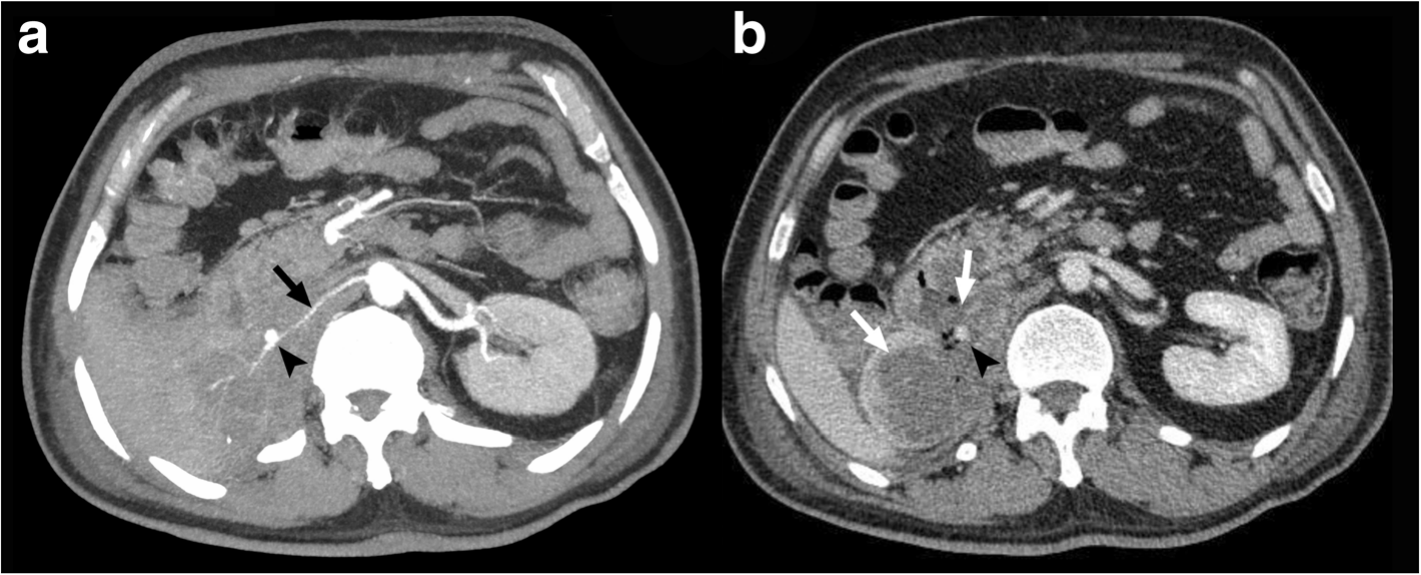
Maximum intensity projection (MIP) reconstruction (a) and thin slice (b) images of contrast-enhanced abdominal CT scan in the arterial phase show a small right renal artery (arrow in a) with a pseudoaneurysm (arrowhead in a and b), and a hypo-enhancing ill-defined mass originating from the right kidney (asterisk in b). There is tumoral erosion and invasion into the adjacent duodenum and remaining renal tissue (white arrows in b)
